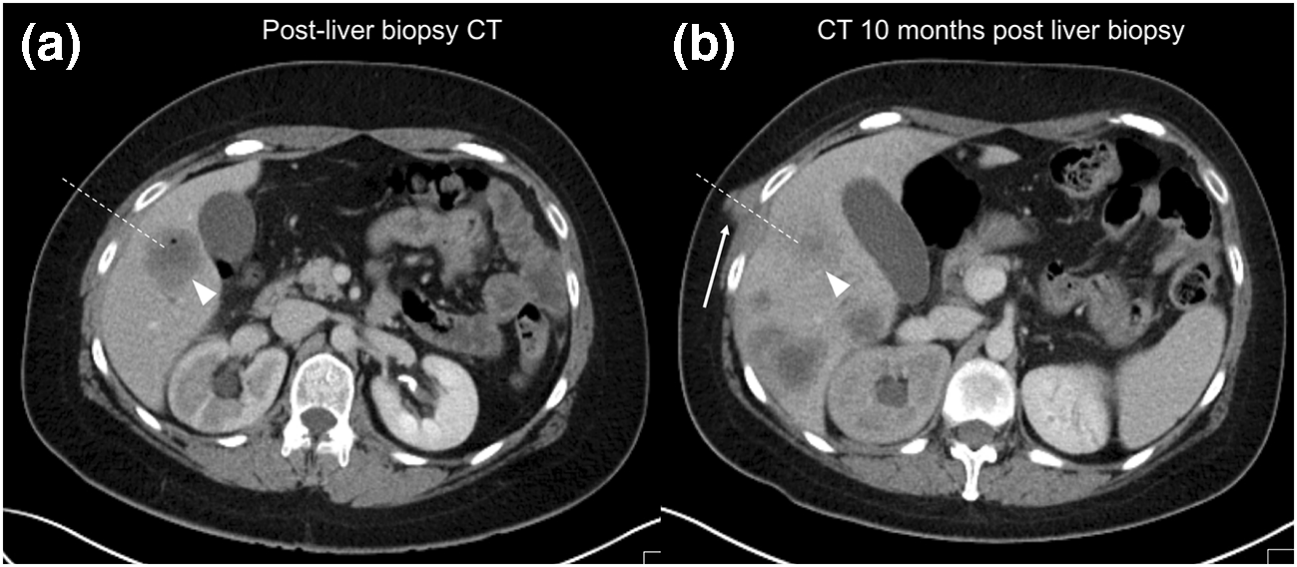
31-year-old man with metastatic colorectal cancer. a Staging CT performed immediately after an ultrasound-guided percutaneous liver biopsy of a lesion in the segment V of the liver (*white arrowhead*, *note gas bubble from biopsy*), which was performed by using a non-coaxial biopsy technique. b On the CT performed 2 months later, there are multiple new lesions in keeping with progressive disease. Furthermore, a new deposit (*white arrow*) is noted in the chest wall, along the needle track (*dotted white line*), indicating tumour seeding
